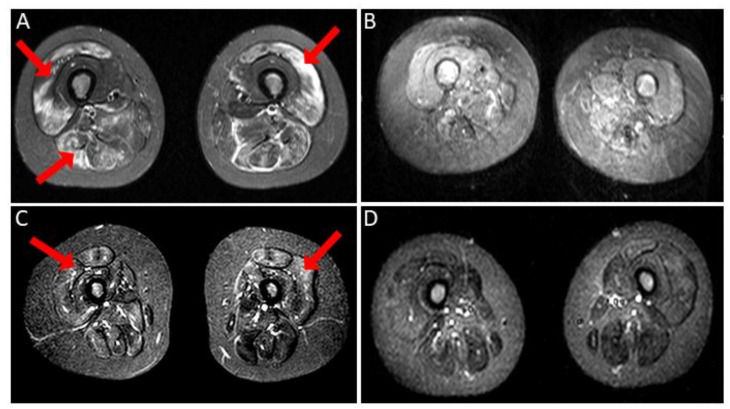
Magnetic resonance imaging (MRI) of both patients using the short tau inversion recovery (STIR) sequence. Patient 1: patchy, symmetrical edema of the thigh muscles at onset (A, arrows) and normalization of the STIR signal as well as marked fatty atrophy of the previously affected thigh muscles after one year of therapy (B). MRI–STIR sequence of Patient 2: patchy signal elevation predominantly in the quadriceps muscles, left more pronounced than the right, 4 months after starting therapy (C, arrows). Reduced signal elevations and atrophy of the quadriceps muscles at 10-year follow up (D).
